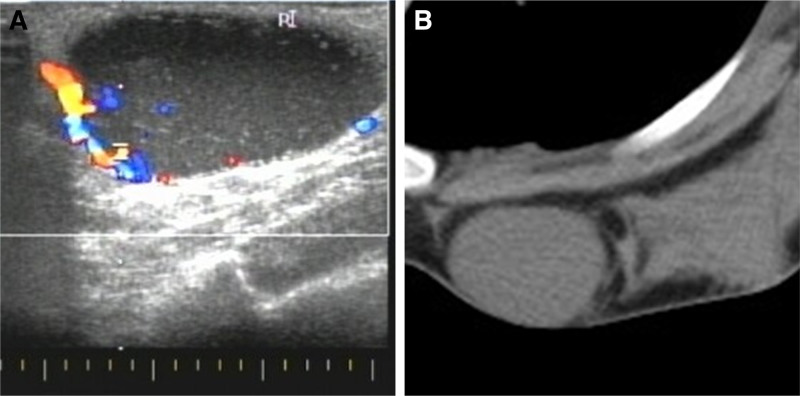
A 15-yr-old woman with a lesion in her right breast that was diagnosed as fibroadenoma. (A) Ultrasonographic images reveal a 43 × 21 mm hypoechoic mass in the lower inner quadrant of the right breast that has clear boundaries, regular morphology, peripheral visible capsule, internal visible striated structure, and slightly abundant blood flow signal in the peripheral area. (B) Computed tomography images of the chest present an iso-density mass in the lower inner quadrant of the right breast, approximately 39 × 29 mm in size.
